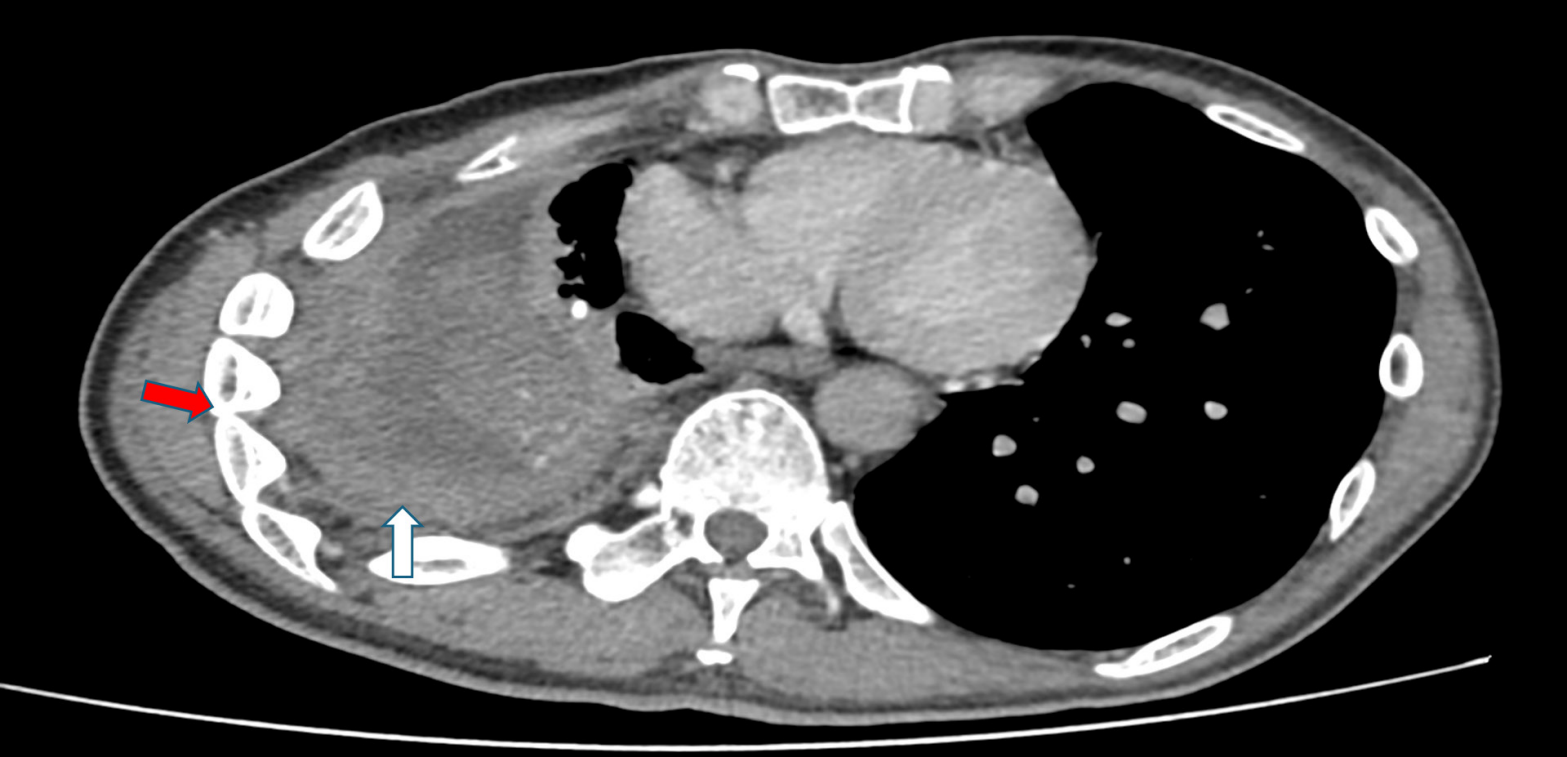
Axial CT showing pleural thickening (white arrow) and rib crowding (red arrow).
